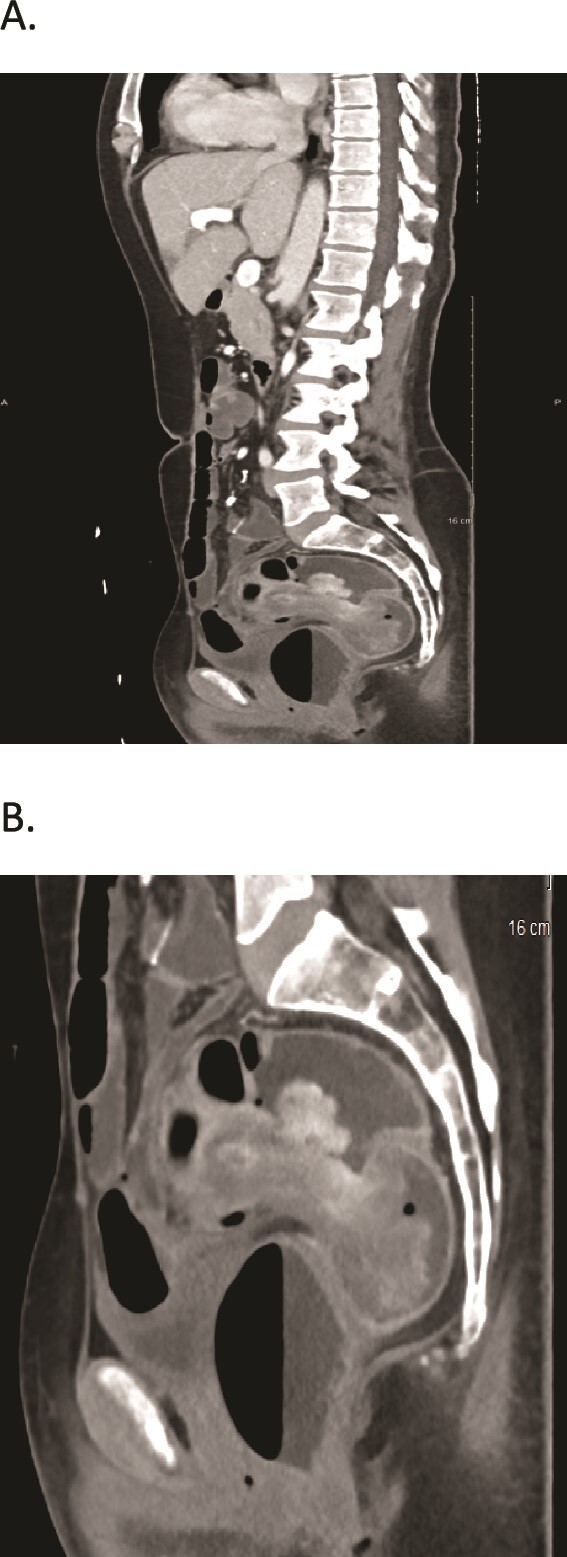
(A, B) CT scans show two large fungating intraluminal sigmoid masses. The largest is 5 × 5.5 × 4.3 (AP, transverse), about 10 cm from the anal verge, and associated with “target sign” in the sigmoidorectal area.
